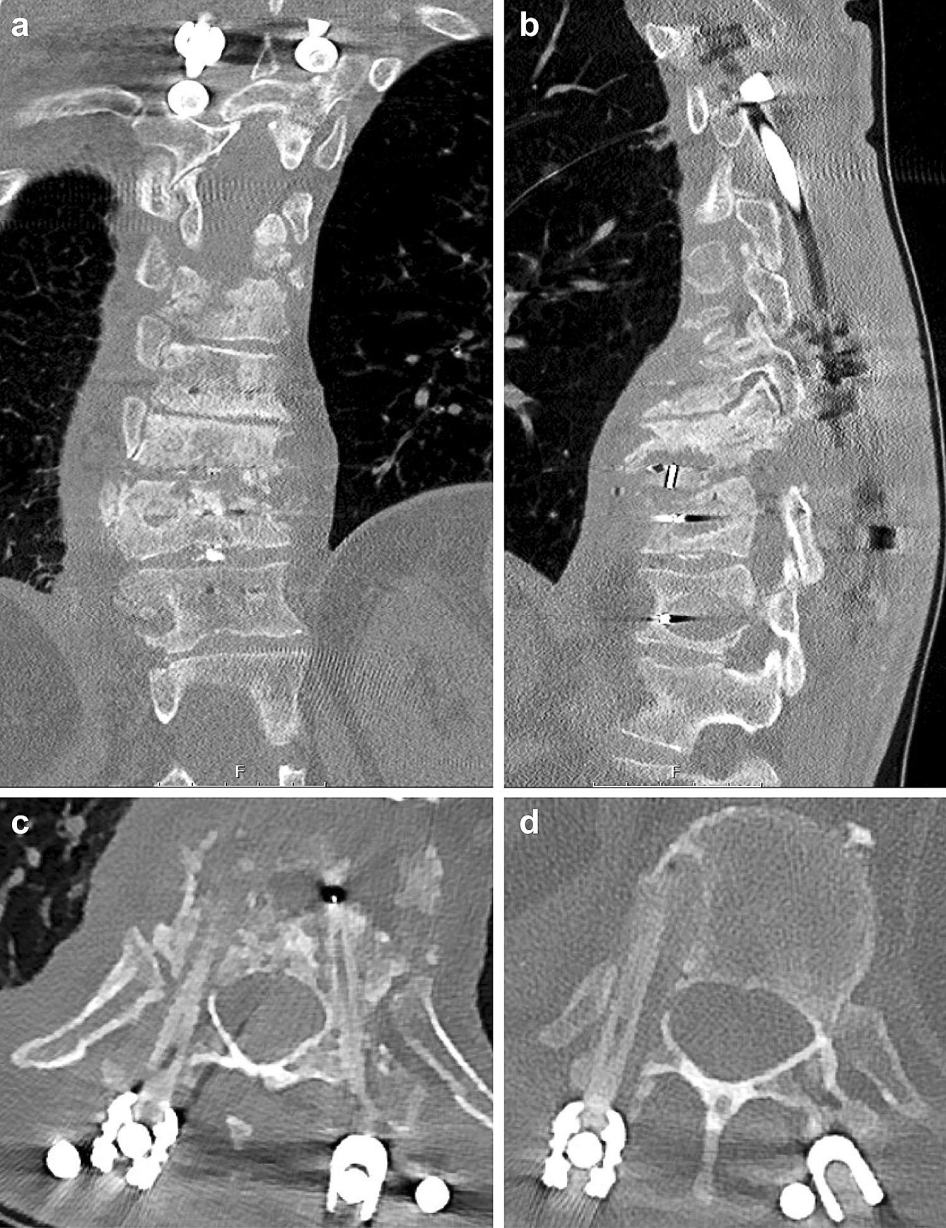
Example 2 of CT-imaging of screw loosening after CFR-PEEK instrumentation. CT-scan of a case of screw loosening after CFR-PEEK instrumentation T5–6–9–10–11–12 involving the affected vertebra on level T11. There a halo signs around the screws of T11 and 12 on the right side as a sign for loosening. (a) coronal plane; (b) sagittal plane, (c,d) axial plane of T11 and T12.
